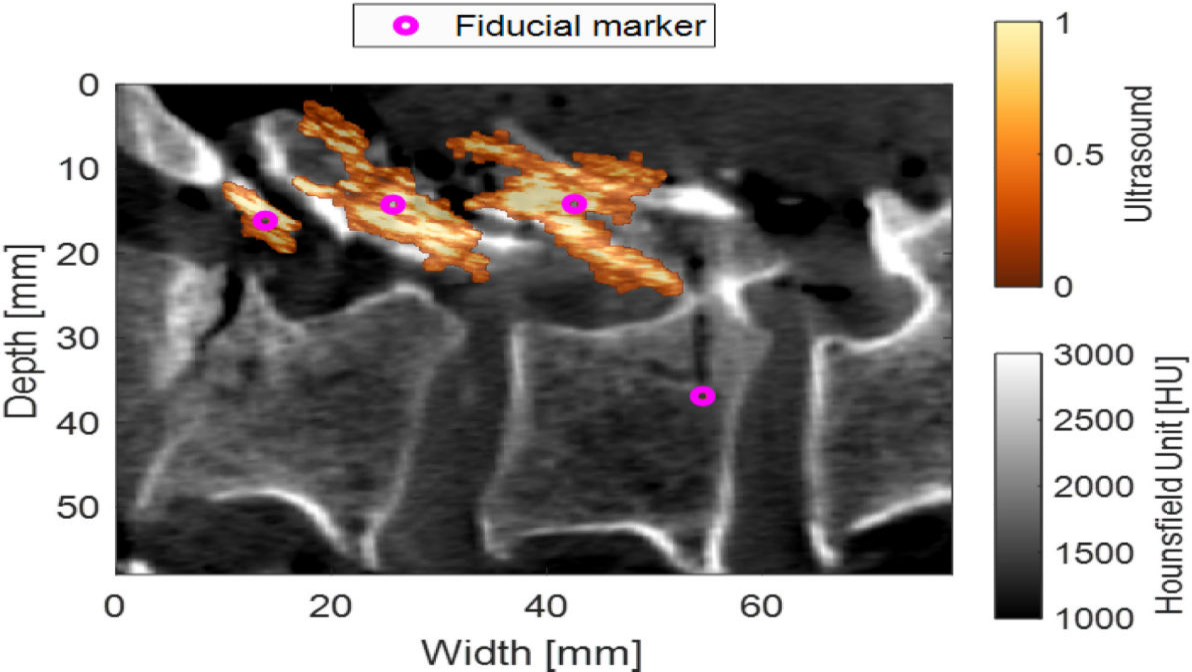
Co-registered ultrasound (color) and CT (grayscale) images using ultrasound and photoacoustic landmarks (magenta) from segmented LW-SLSC images.
